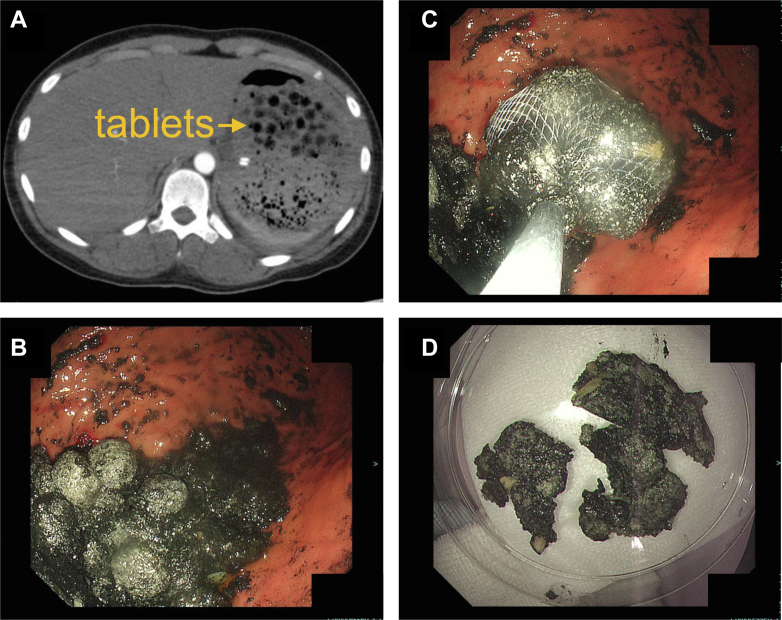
Remaining Tablets on Computed Tomography and Endoscopic Removal Performed on Day 111 (A) CT showed undissolved tablets in the stomach. (B to D) They were endoscopically removed.
